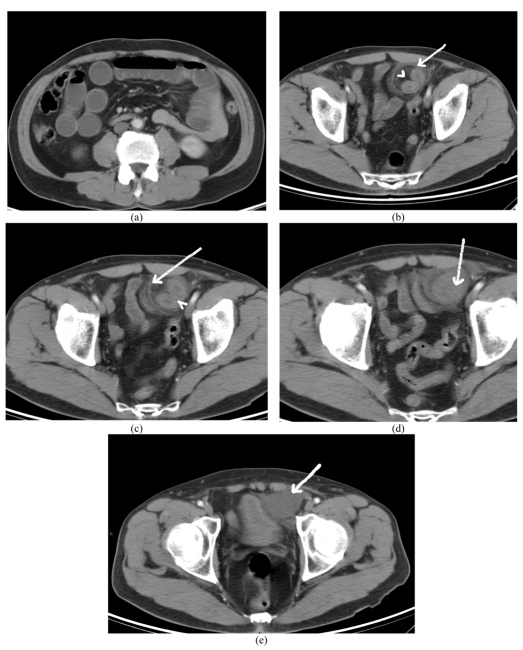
(a) CT scan of the abdomen demonstrating dilated small bowel loops suggestive of bowel obstruction. (b) The hernial sac [arrow] containing thickened bowel loop and mesenteric fat [arrowhead] is seen in the properitoneal space, superior to the left inguinal region. (c) A fibrous constriction band is seen around the neck of the hernial sac [arrow] .Note enhancement of bowel wall [arrowhead]. (d) The inferior aspect of the hernial sac containing bowel [arrow]. (e) Fluid in the hernial sac [arrow]. Wall enhancement of the bowel loop and fluid in the hernial sac suggest incarceration and strangulation.
